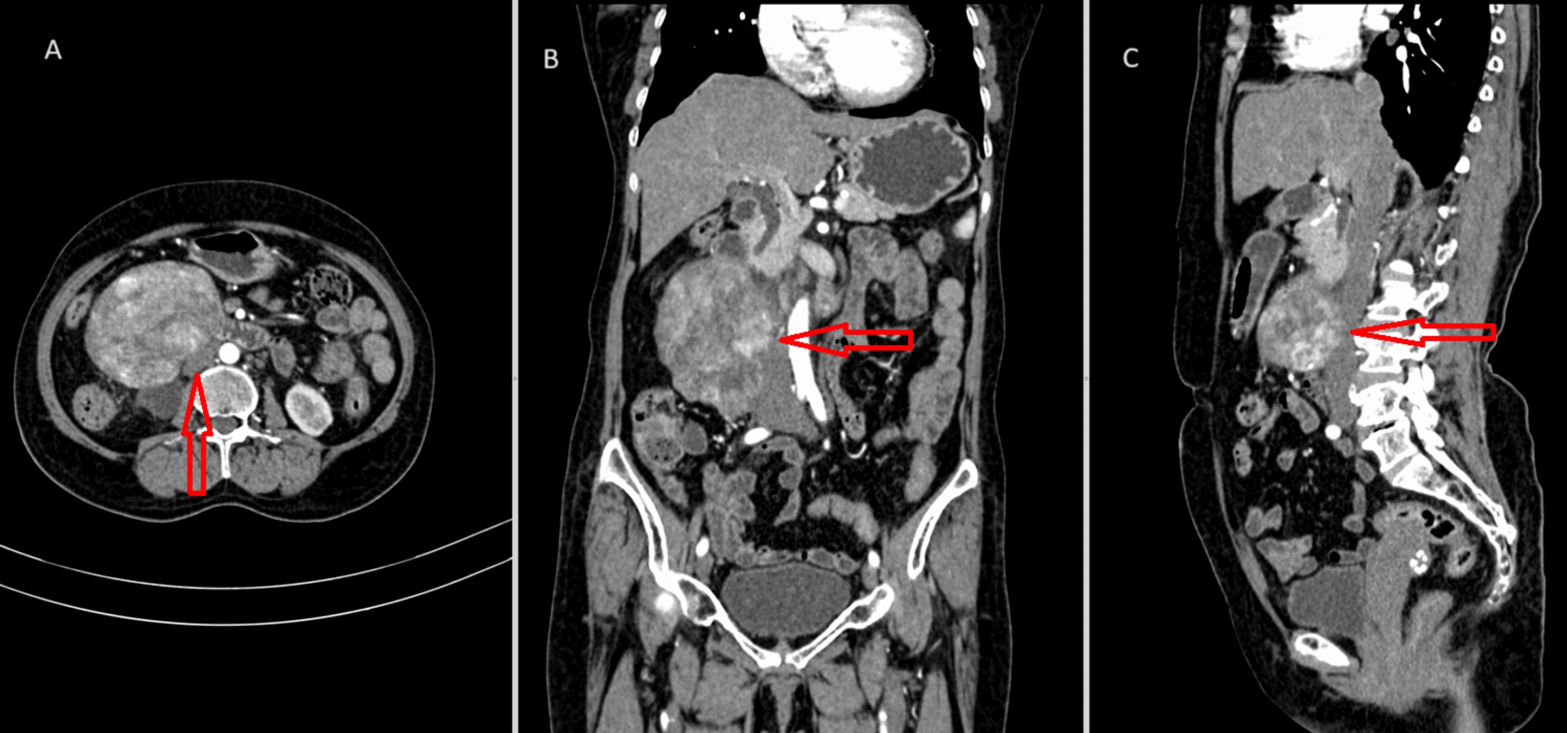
CECT of the abdomen in axial (A), coronal (B), and sagittal (C) sections, with red arrows showing lesions causing mass effect on IVC CECT: contrast-enhanced computed tomography; IVC: inferior vena cava
